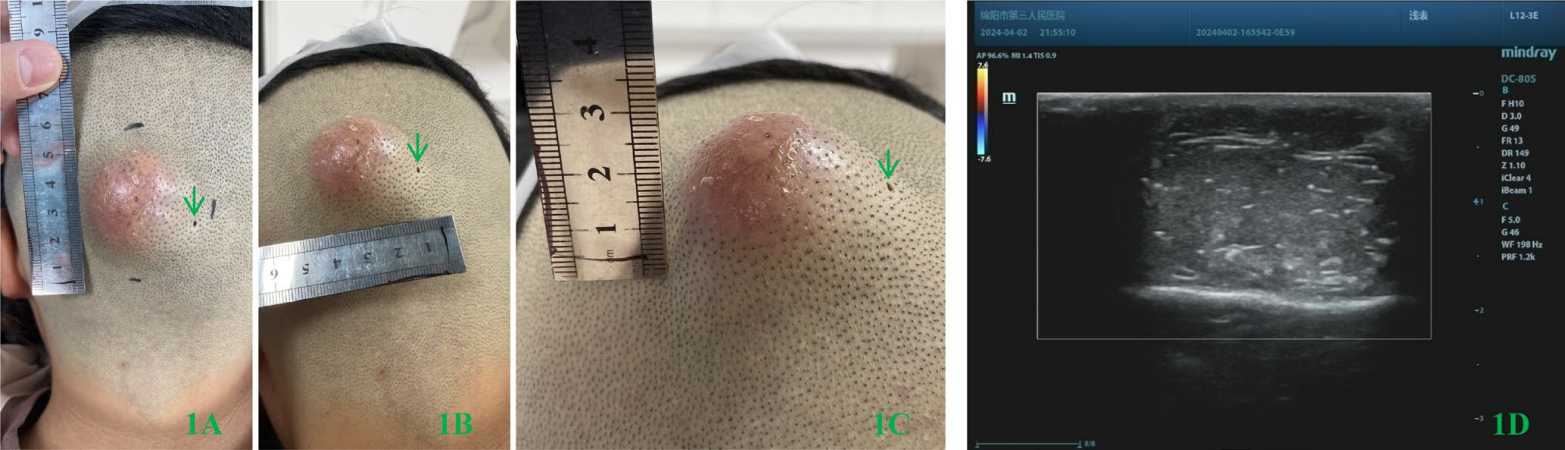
(A–C) The length, width, and height of the lump was 4.5 cm, 4.5 cm, and 3 cm, respectively. A small skin opening adjacent to the lump as indicated by the green arrow. (D) Color ultrasound indicated a hypoechoic area without blood flow signal in occipitalia scalp.
